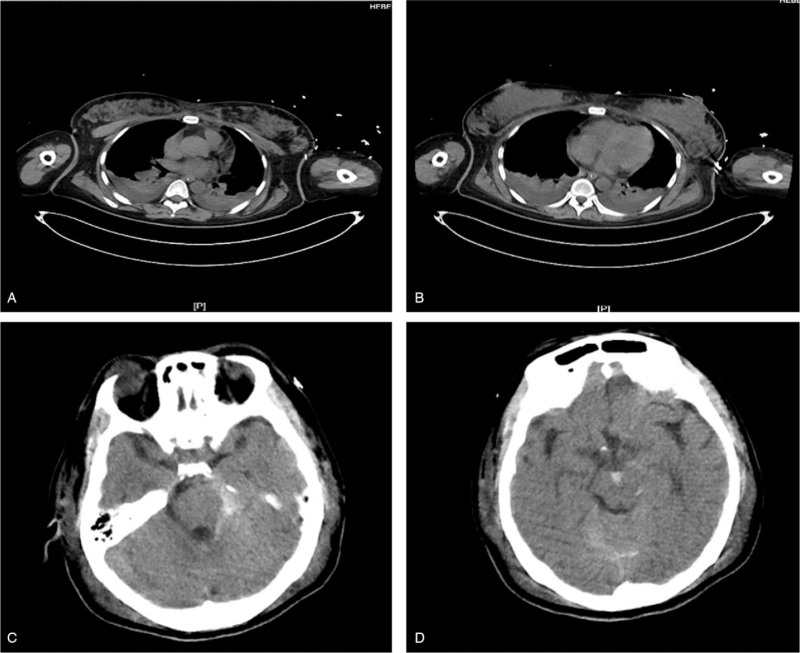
CT scans of the chest and brain in the ICU on day 5. (A) & (B) CT scans of the chest showed the left pleural effusion was significantly reduced. (C) & (D) CT scans of brain showed the area of subarachnoid hemorrhage was not enlarged. CT = computed tomography, ICU = intensive care unit.
